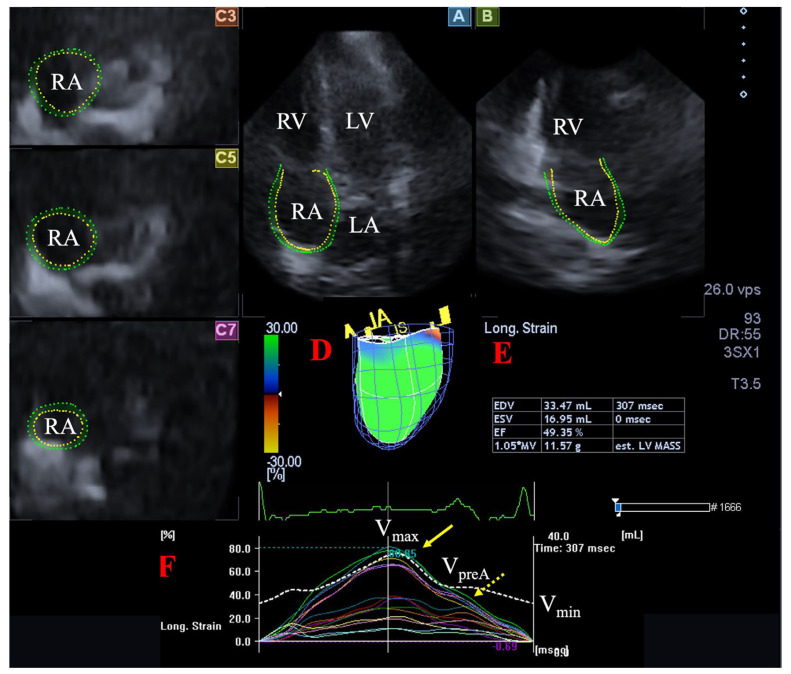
Assessment of the right atrium (RA) is presented extracted from a three-dimensional (3D) echocardiographic full-volume dataset: apical four-chamber view (A), apical two-chamber view (B), short-axis views at basal (C3), midatrial (C5), and superior (C7) RA levels. Virtual 3D cast of the RA (D), calculated RA volumes (E) and RA volume changes (dashed white line), and global and segmental RA (longitudinal) strain changes (white and coloured lines) over time (F) are also presented. Yellow arrow represents peak RA strains (dashed yellow arrow represents RA strains at atrial contraction, not examined). Abbreviations. LA = left atrium, LV = left ventricle, RA = right atrium, RV = right ventricle, V_max_ = end-systolic maximum RA volume, V_min_ = end-diastolic minimum RA volume, V_preA_ = preatrial contraction RA volume.
